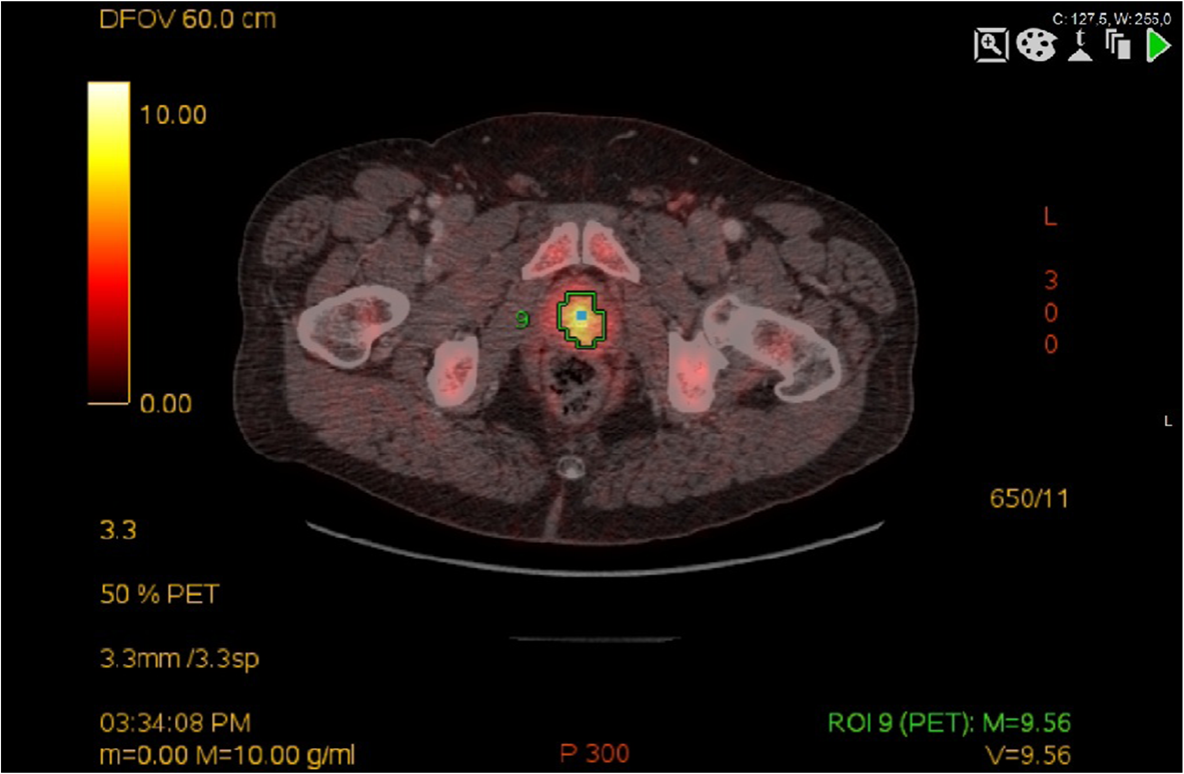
Increased acetate uptake (SUV
